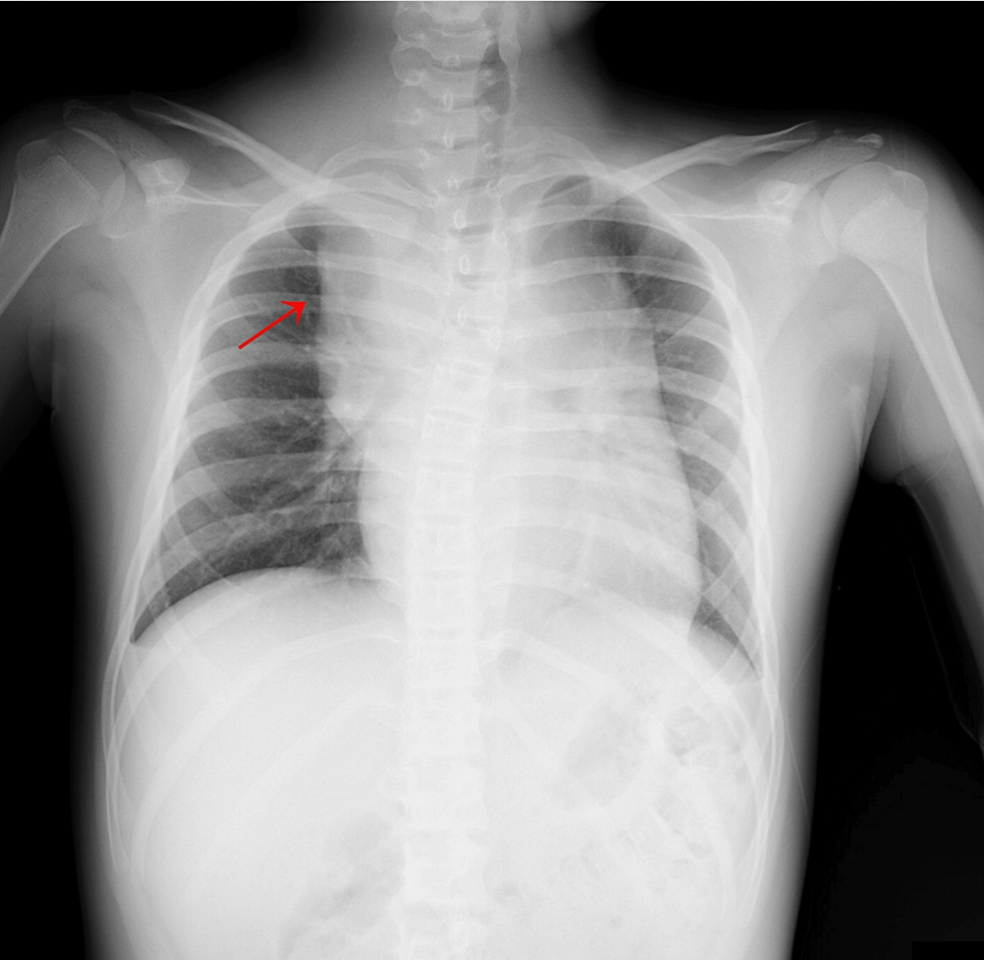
Chest X-ray on arrival Chest X-ray showing an enlarged superior mediastinum (red arrow).
